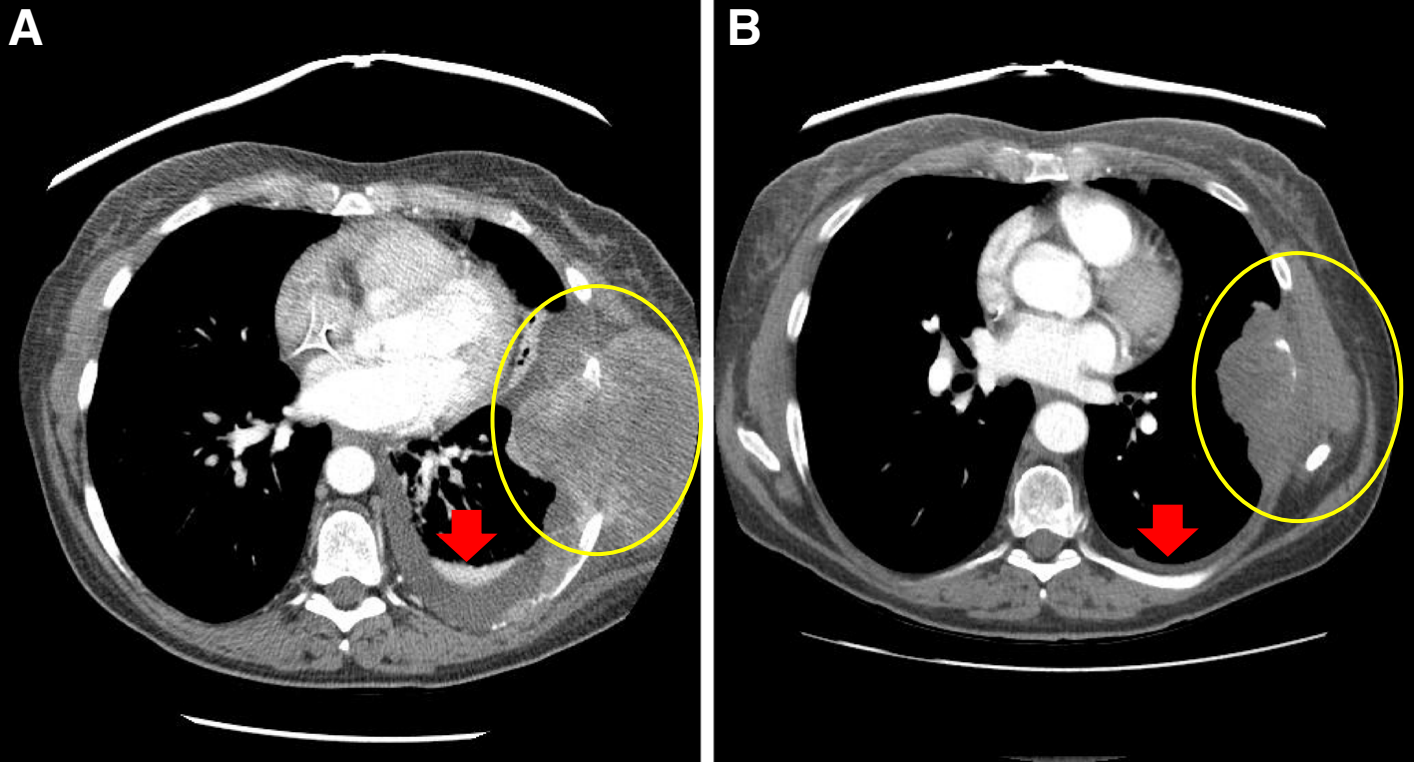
Imaging studies pre and post- treatment. A: Pre-treatment CT scan of the Chest shows left chest wall tumor invading the ribs and left sided pleural effusion. B. Post-treatment CT scan of the Chest shows a decrease in size of the tumor and decrease in pleural effusion.
